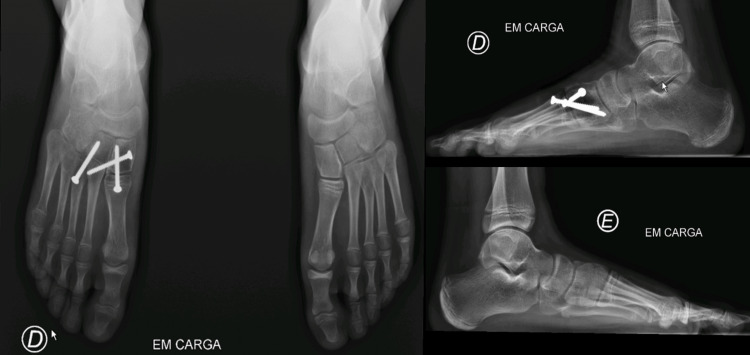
Weight-bearing bilateral X-rays: 4 months postoperative.
